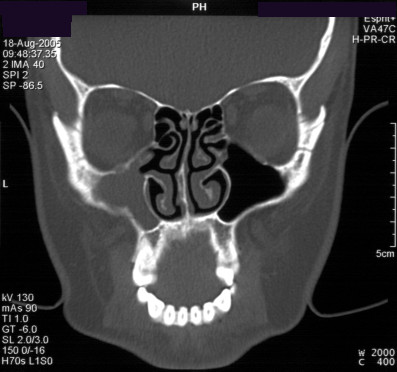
Preoperative CT scan of the sinuses. Coronal view demonstrating opacified left maxillary sinus.
